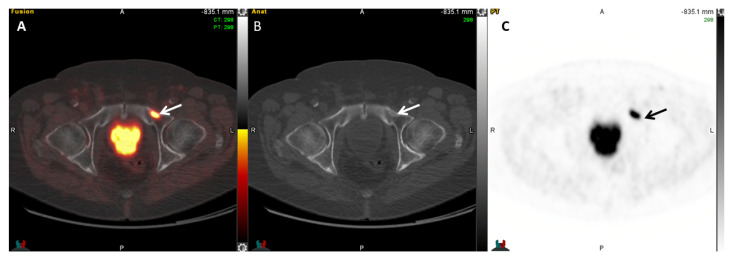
A 74-year-old man with biochemically recurrent prostate cancer with serum PSA of 2.7 ng/mL. Fused axial PET/CT (A), axial CT (B), and PET (C) images demonstrate a focus of increased ^64^Cu-PSMA I&T uptake in the left superior pubic ramus (SUVmax 16.2, arrows), compatible with a bone metastasis.
